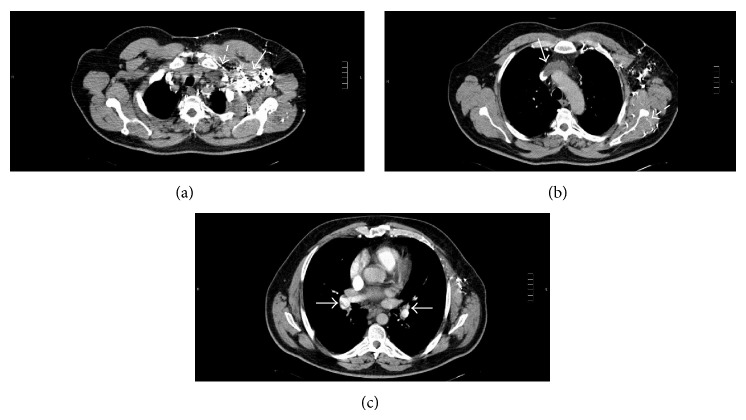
(a) Chest computed tomography angiogram. Thrombotic obstruction of the left brachiocephalic vein (dashed arrow) with multiple collateral vessels near the left clavicle and in the upper left limb (full arrow). (b) Chest computed tomography angiogram. Extension of the left brachiocephalic vein thrombus into the superior vena cava (full arrow). Collateral vessels are seen adjacent to the left clavicle and in the upper left limb (dashed arrow). (c) Chest computed tomography angiogram. Arterial thromboemboli in the pulmonary arteries (full arrows).
